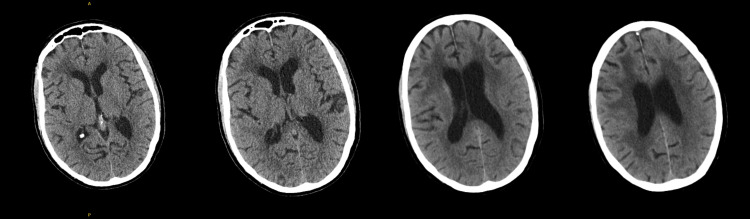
CT head showing no acute pathology Non-contrast axial CT images of the brain show no evidence of acute intracranial hemorrhage or large territorial infarction.
